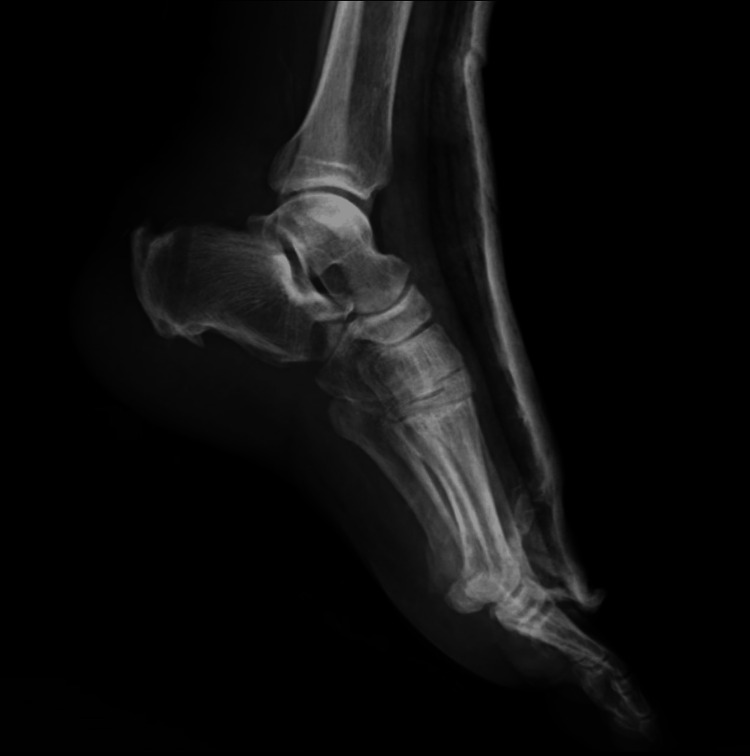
Post-operative X-ray following flexor hallucis longus transfer with tendo-Achilles augmentation Case 1
